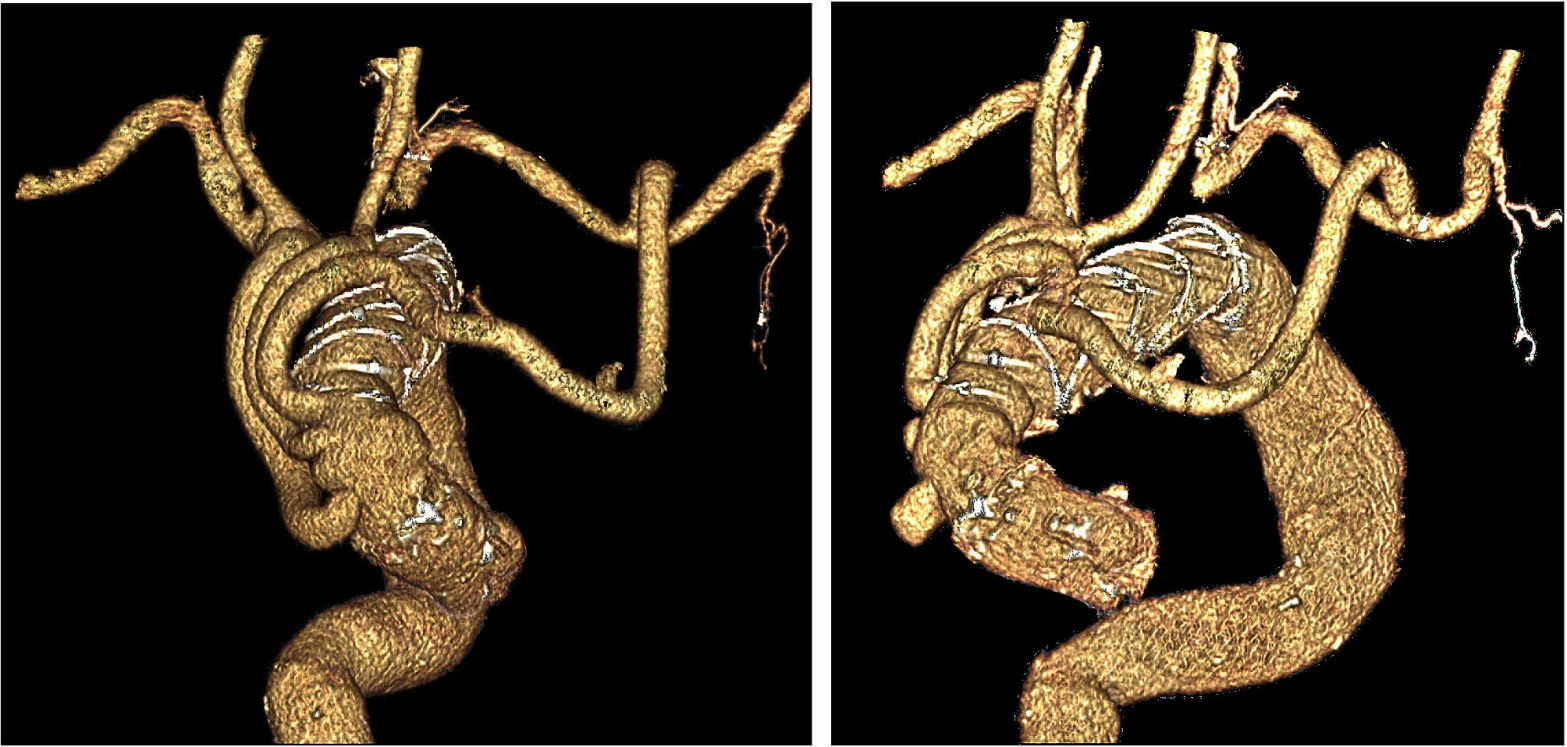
Tridimensional reconstruction of postoperative CT-scan showing complete coverage of the arch and debranching of SAVs from zone 0
